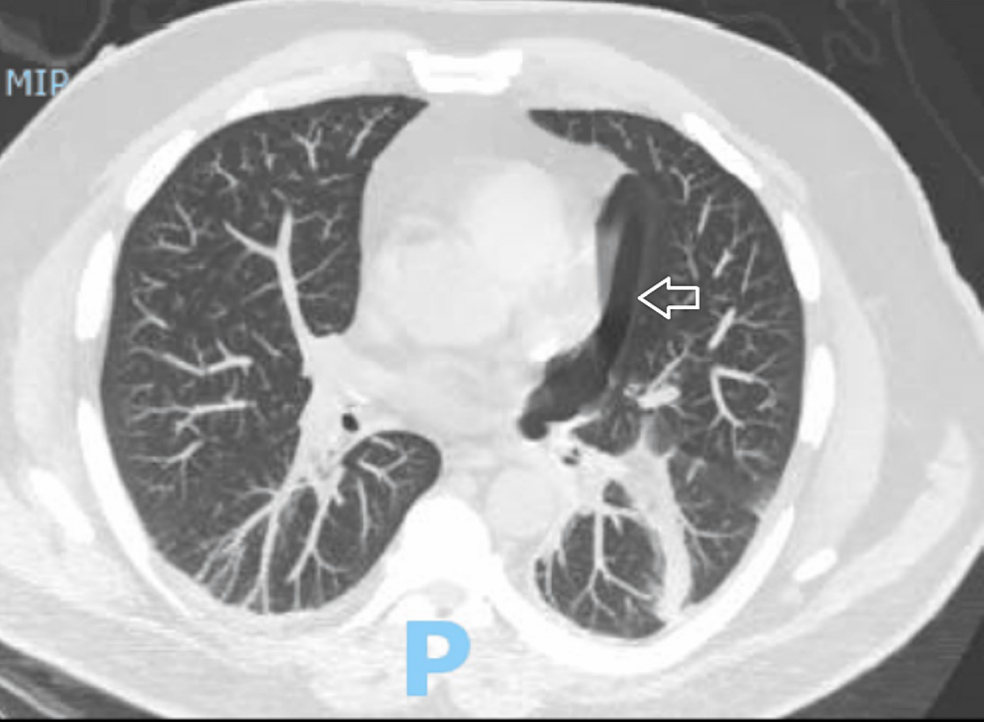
Chest x-ray showing left pneumothorax (arrow) with air gap measuring 7.6 cm.
